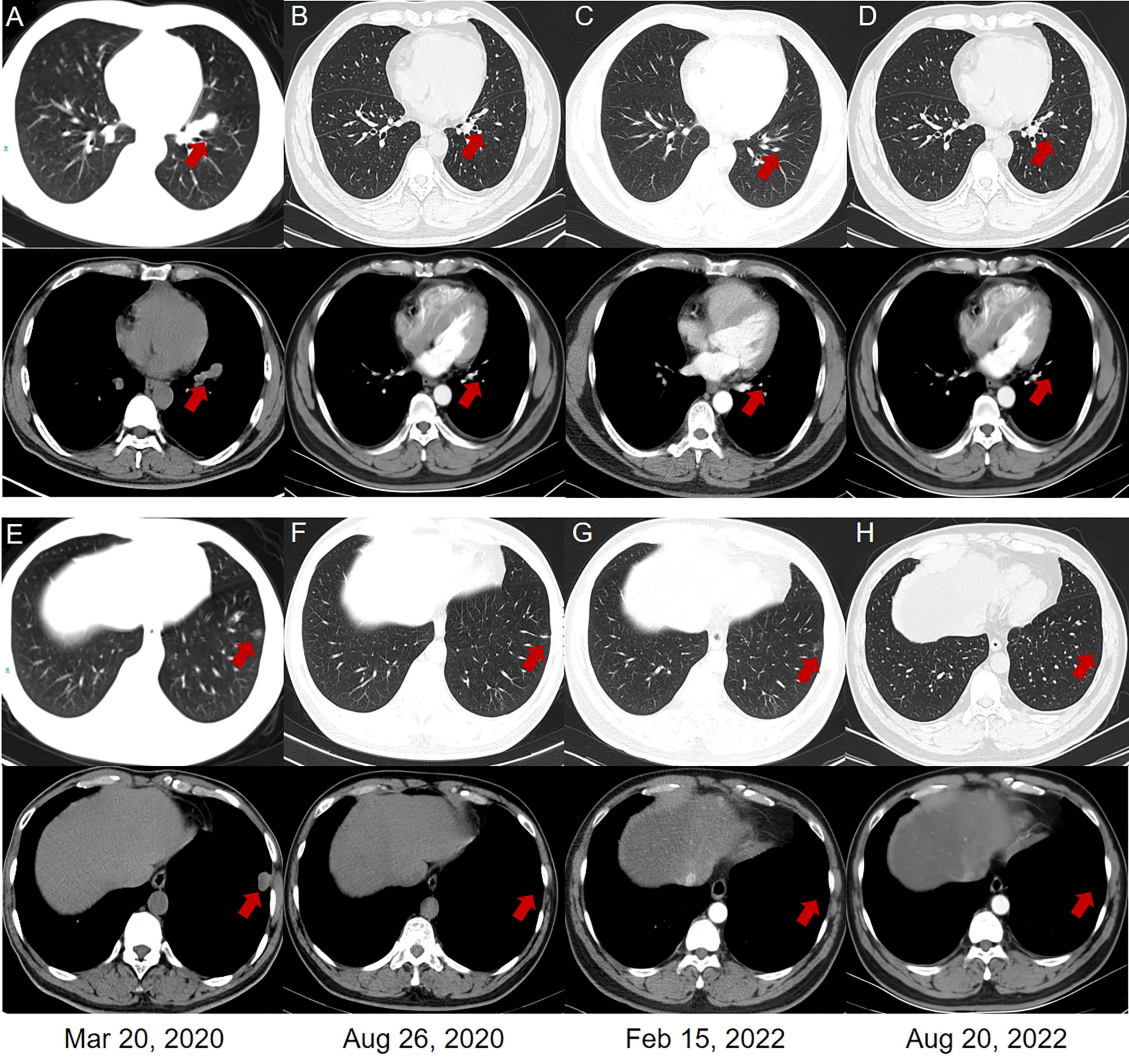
Chest computed tomography (CT) scans. (A, E) In March 2020, multiple metastases of lung and mediastinal window were observed. (B, F) In August 2020, a PR was revealed in the lung and mediastinal window of the patient after the combined treatment of pembrolizumab and anlotinib. The patient obtained a PR (C, G) and CR (D, H) in the lung and mediastinal window after the monotherapy of pembrolizumab.
